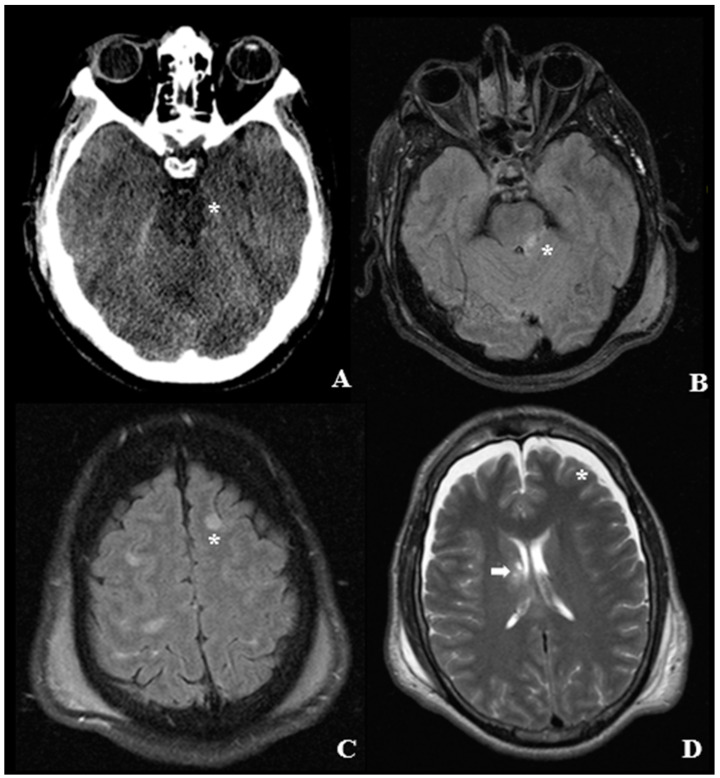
Radiological findings in a case of DAI—(A) First Computed tomography (CT) scan disclosing the absence of Ambiens Cistern and a slight hypodensity of the transition zone between Mesencephalon and Pons (asterisk). (B,C) Flair sequence late MRI disclosing the diffuse involvement of the subcortical with matter and of the Brachium Pontis (asterisks). (D) T2 weighted MRI scan disclosing the late atrophic changes of the Supratentorial cerebrum, with a bilateral Subdural Hygroma (asterisk). The arrow highlights a neural injury at the transition between the body of the Caudate Nucleus and the Internal Capsule.
